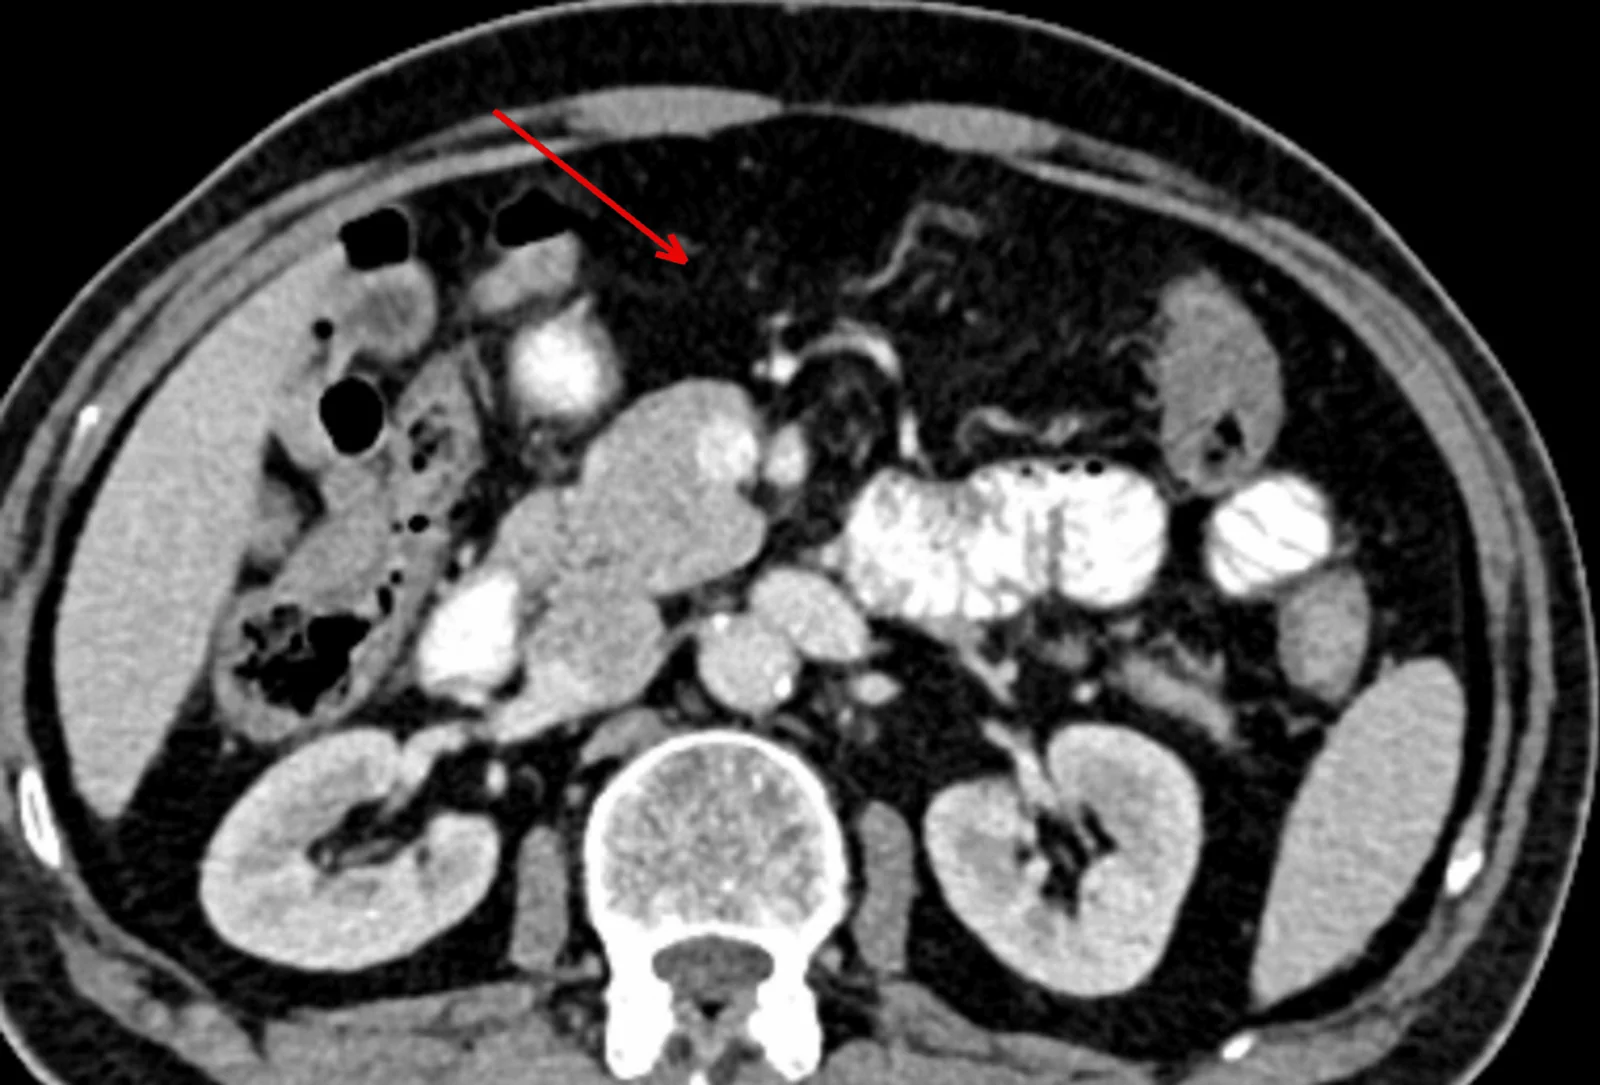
Venous-phase contrast-enhanced abdominal CT demonstrating a bulky pancreatic head and uncinate process (arrow) with a patent portal venous system
